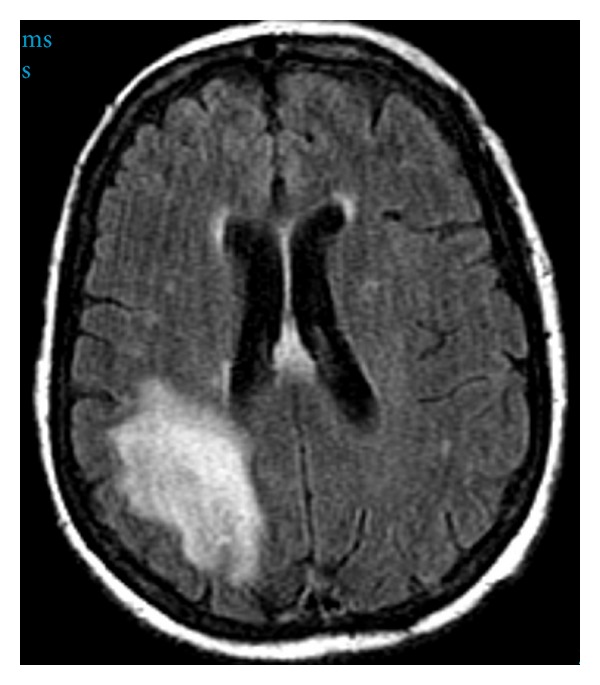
Axial T2-FLAIR image shows increase in signal intensity in the subcortical white matter involving the U-fibers in the right parietal lobe.
